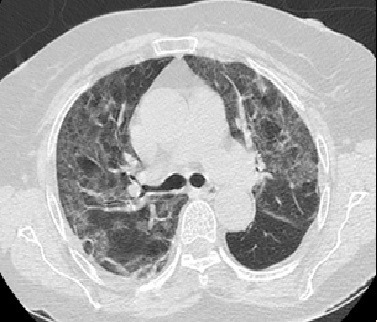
Axial computed tomography image of the chest, showing bilateral ground glass signs.
